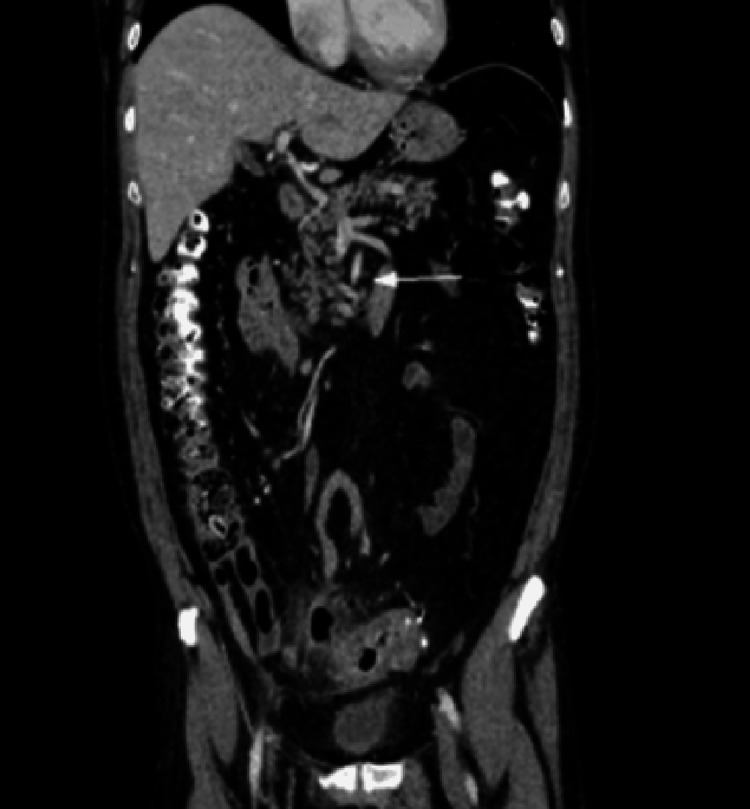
Abdomen CT scan with contrast. White arrow indicates inferior mesenteric vein without connection with the splenic vein; it flows directly into the portal vein.
